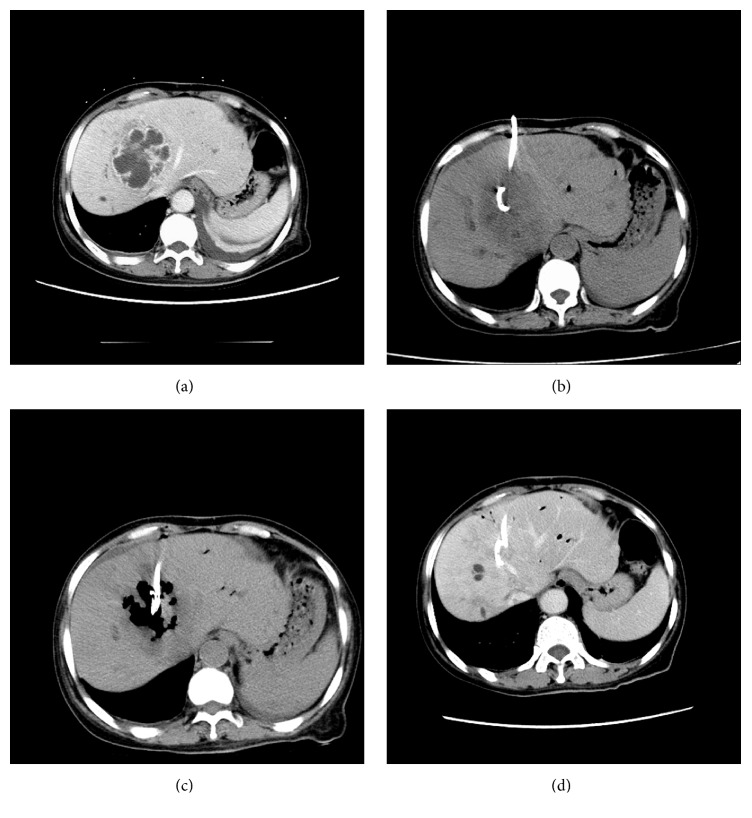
A 49-year-old male presented with fever and right back pain for one week. (a) A lager multiloculated liver abscess in the right hepatic lobes was shown by enhanced CT, and small amount of fluid can be seen in the left chest. (b) Catheter was placed under CT guidance, without injection of oxygen-ozone gas. (c) Three-week follow-up showing that abscess was not fully absorbed. (d) Abscess was fully absorbed after eight weeks.
